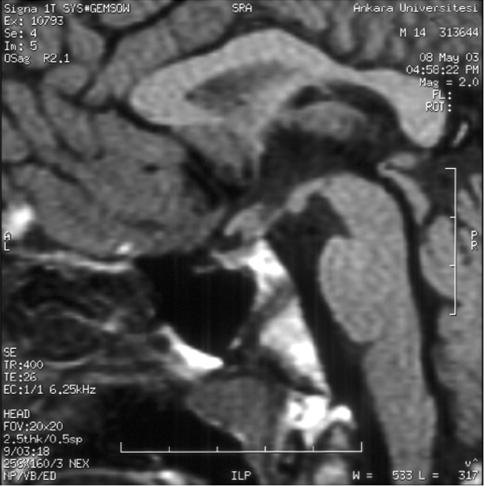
MRI at admission: A 5 mm infundibular thickness, absence of posterior hypophyseal bright spot
